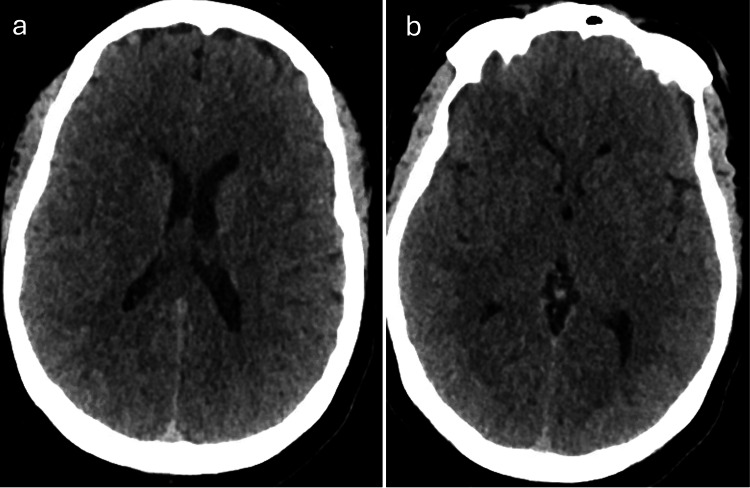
Axial non-contrast brain CT scans showing no detectable abnormalities in the parietal lobe (a) and occipital lobe (b)
